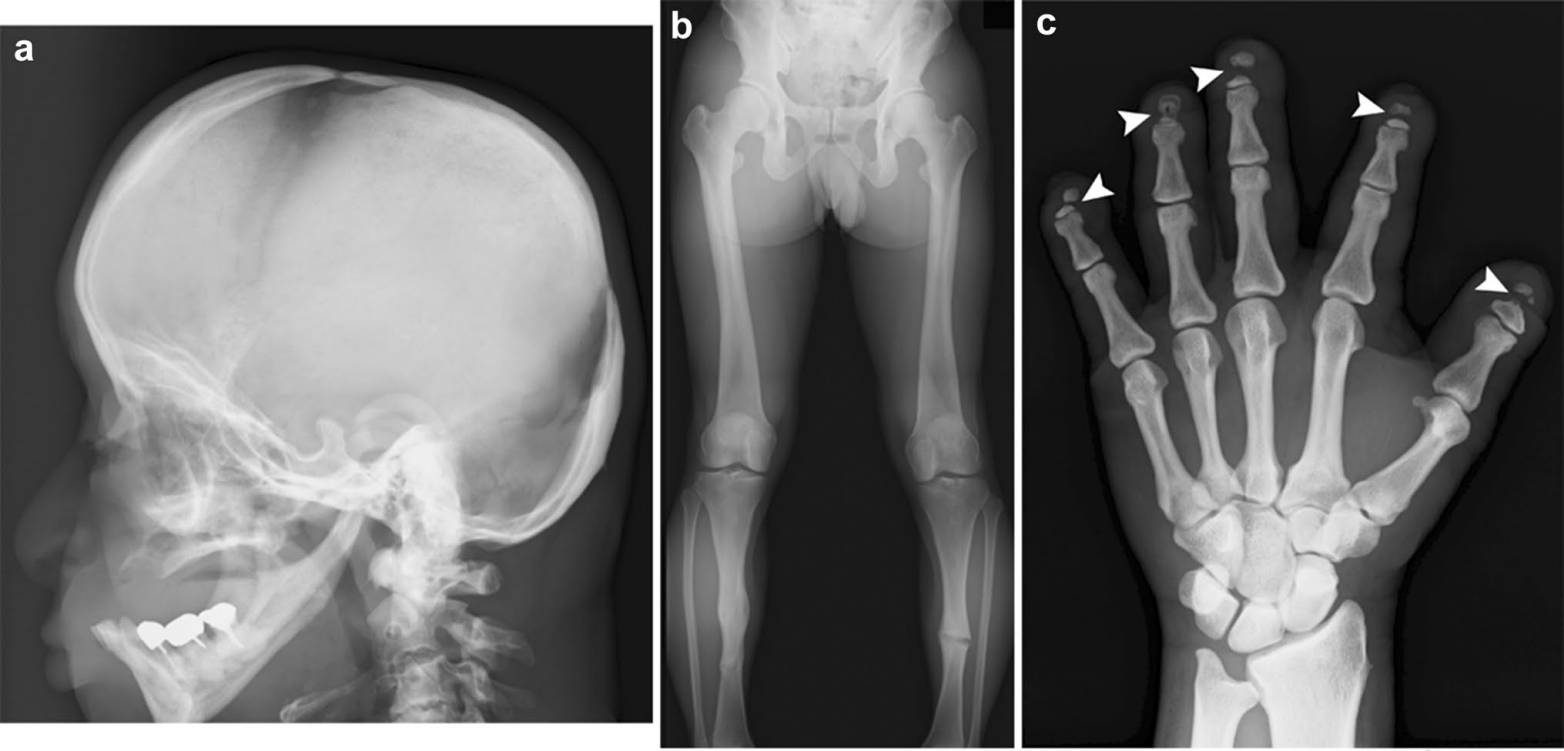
a–c A young adult with pyknodysostosis. Radiographs show craniofacial sclerosis with widely opened fontanels, severe micrognathia with an obtuse mandibular angle, and edentulous state due to repeated dental infections as well as generalized increased bone density with a preserved corticomedullary differentiation and trabecular pattern, healed fractures of both tibiae and c severe acroosteolysis (arrowheads)
